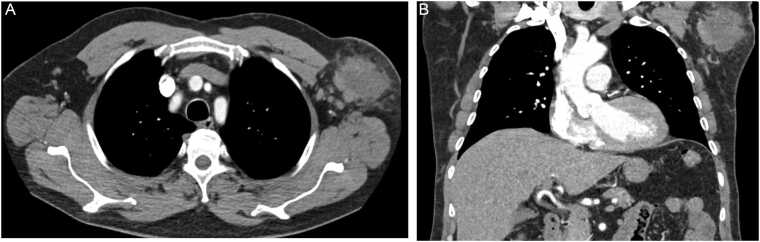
a,b: Computed tomography (CT) scans of the axilla suggesting a 6–7 cm lymphoma in the left axilla.
